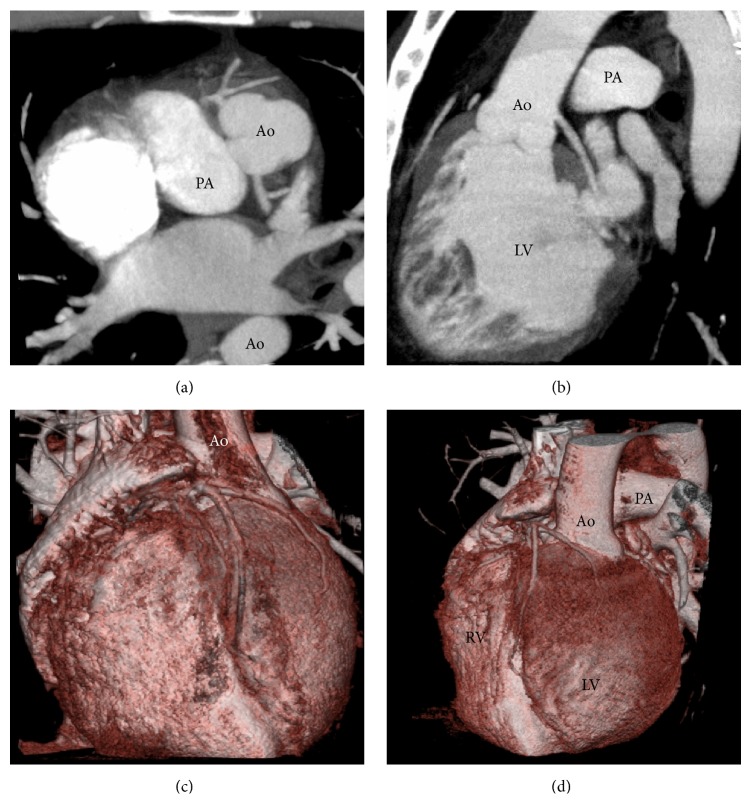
Paraxial (a) and parasagittal (b) maximum intensity projection reconstruction from cardiac CT scan, demonstrating the anomalous site of the coronary ostia as well as the malposition of the great arteries, confirmed by 3D volume-rendered reconstruction ((c), (d)).
